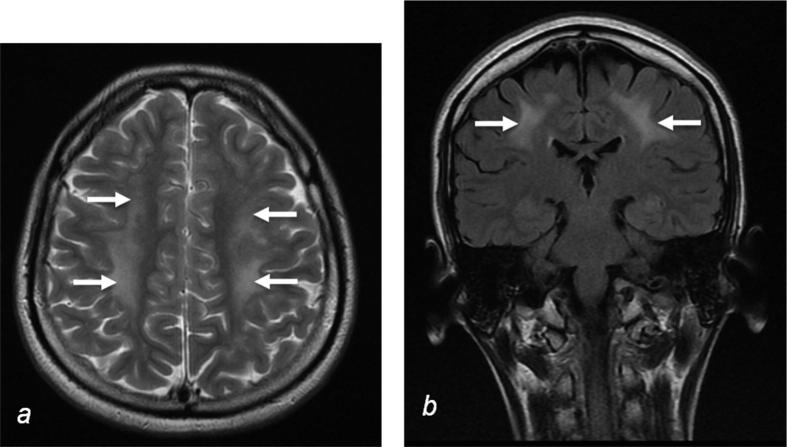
MRI findings. Cranial MRI demonstrates confluent high signal (arrows) in white matter of the frontal and parietal lobes in axial (a, T2 weighted) and coronal (b, FLAIR) sequences, suggestive of leukoencephalopathy.
